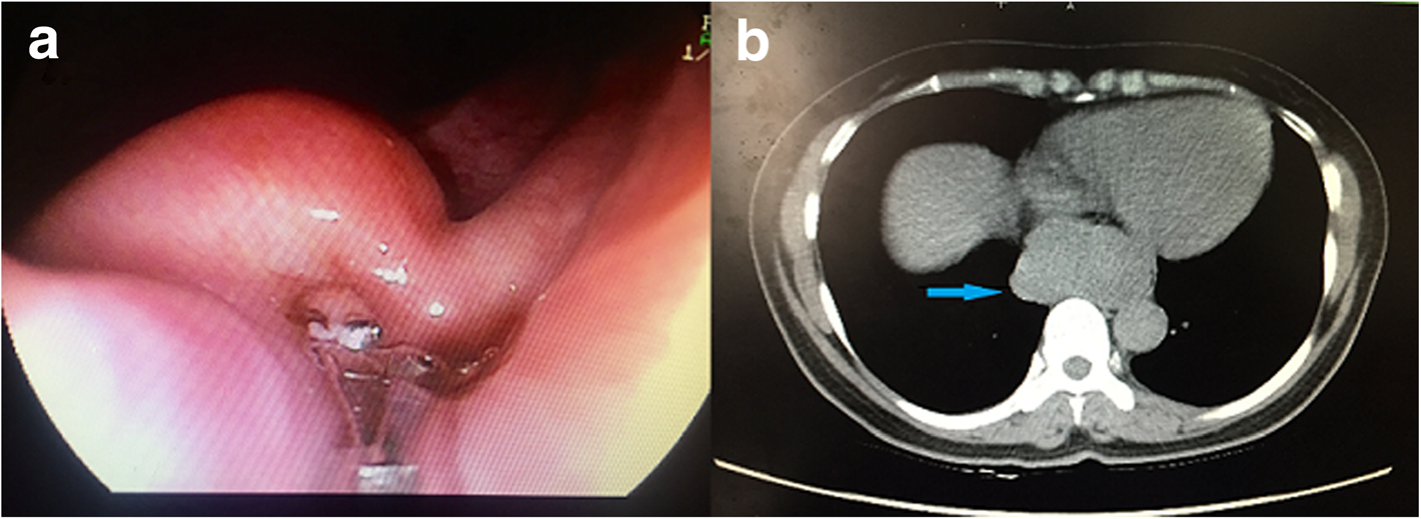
Preoperative imaging of the large esophageal SMT. (a) Upper endoscopy revealed a submucosal tumor 70 mm in length that was 30 cm from the incisor with an intact overlying mucosa. (b) CT scan of the chest revealed a 69 × 36 mm homogeneous mass in the distal esophageal wall (arrow)
